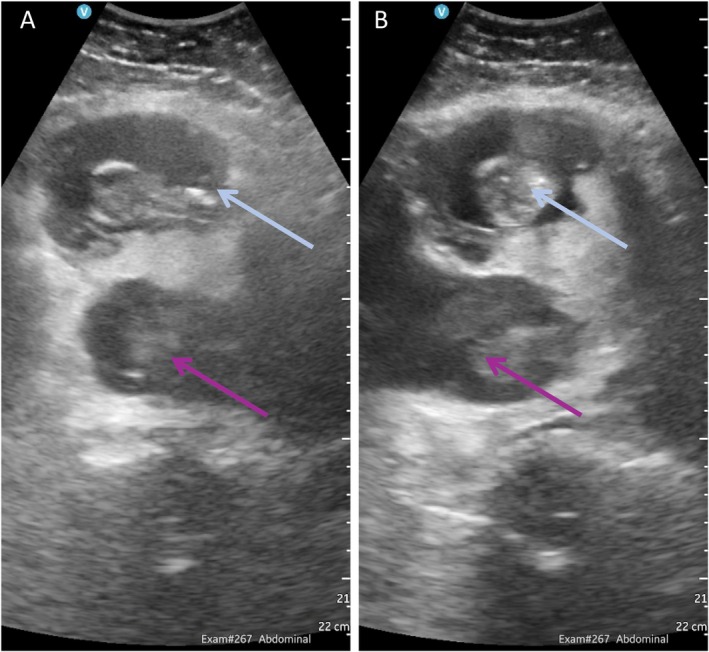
Point of care ultrasound images. Sagittal (A) and transverse (B) images of uterus (purple) and gestation sac (blue) on point of care ultrasound.
